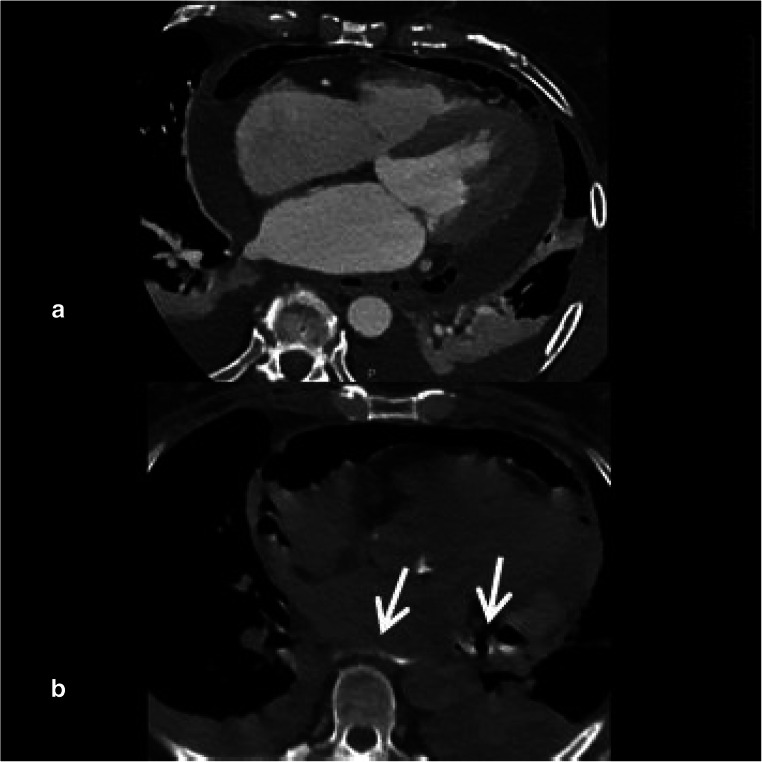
Panel a: Left atrial CT angiography rules out atrio-esophageal fistula as neither air bubble in the cardiac chambers nor contrast extravasation is visible. On the other hand, pneumopericardium is present. Panel b: Swallow test with gastrografin under CT imaging shows that the contrast agent enters the pericardium (arrows) but does not enter the left atrium; thus, diagnosis is a pericardial-esophageal fistula
